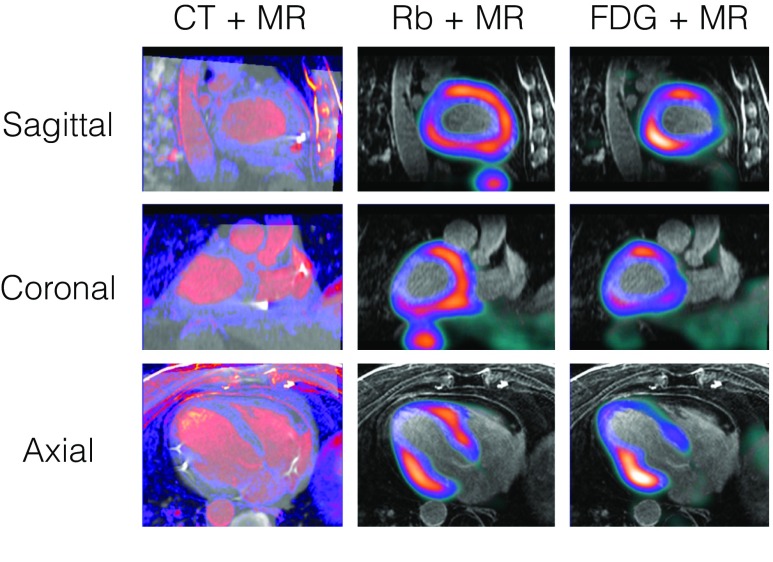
Image fusion of separately acquired PET/CT and CMR images in a patient with cardiac sarcoidosis. CT images from a hybrid PET-CT scanner were used to fuse the PET images (color) with MR images (B&W). Shown are fused CT and CMR images (left), fused ^82^Rb perfusion PET and CMR (middle), and fused ^18^FDG inflammation PET and CMR (right) studies. Image fusion and figure provided courtesy of Mary Germino, PhD, Yale University
